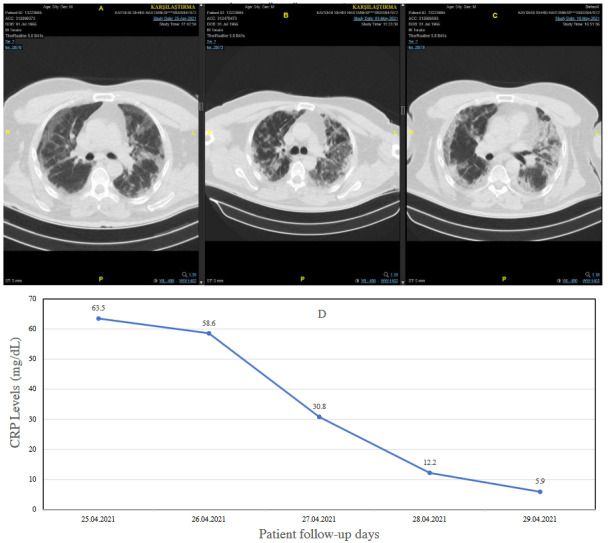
A sample for sepsis/septic shock and MODS associated with COVID-19. A 54-year-old male with type 2 diabetes complained of shortness of breath (SaO2: 77) and weakness and was monitored in the ICU. A) Chest CT at the time of admission (CT) showed a typical ground glass image that corresponded to a COVID-19 peripheral weighted bilateral multifocal patchy. B) Chest CT on day nine at hospitalization. C) Chest CT on day 23 and out of the hospital with an oxygen condenser. D) CRP course of the patient.
